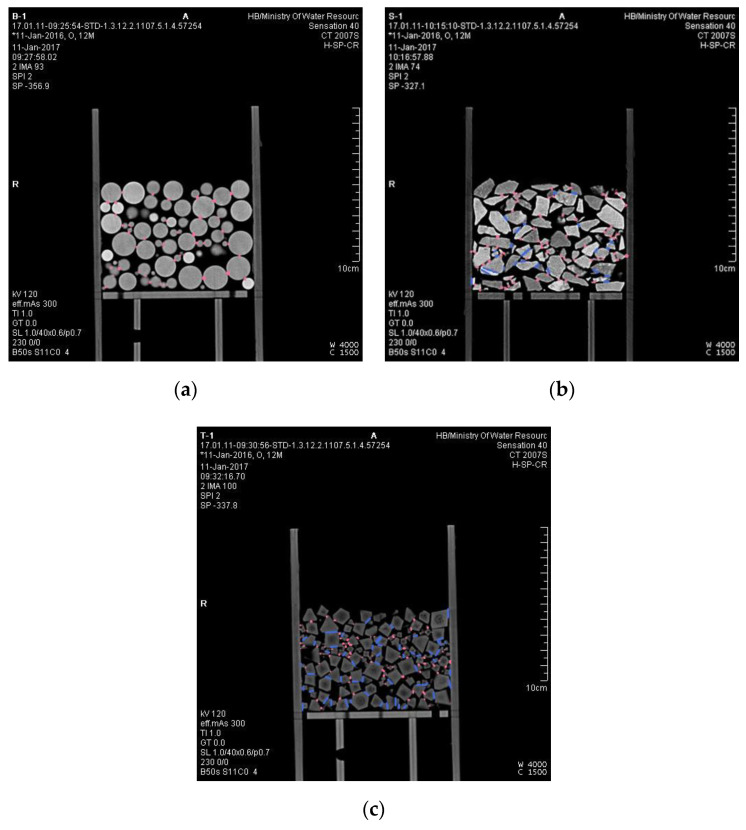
Contact markers on CT images. (a) CT middle section of B1. (b) CT middle section of S1. (c) CT middle section of O1.
